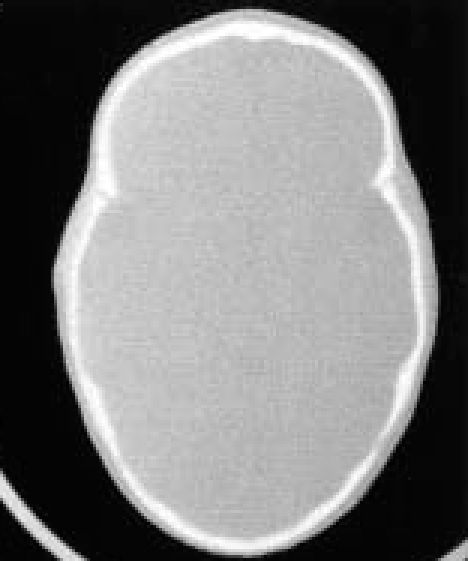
CT native bone window: pathognomonic aspect
